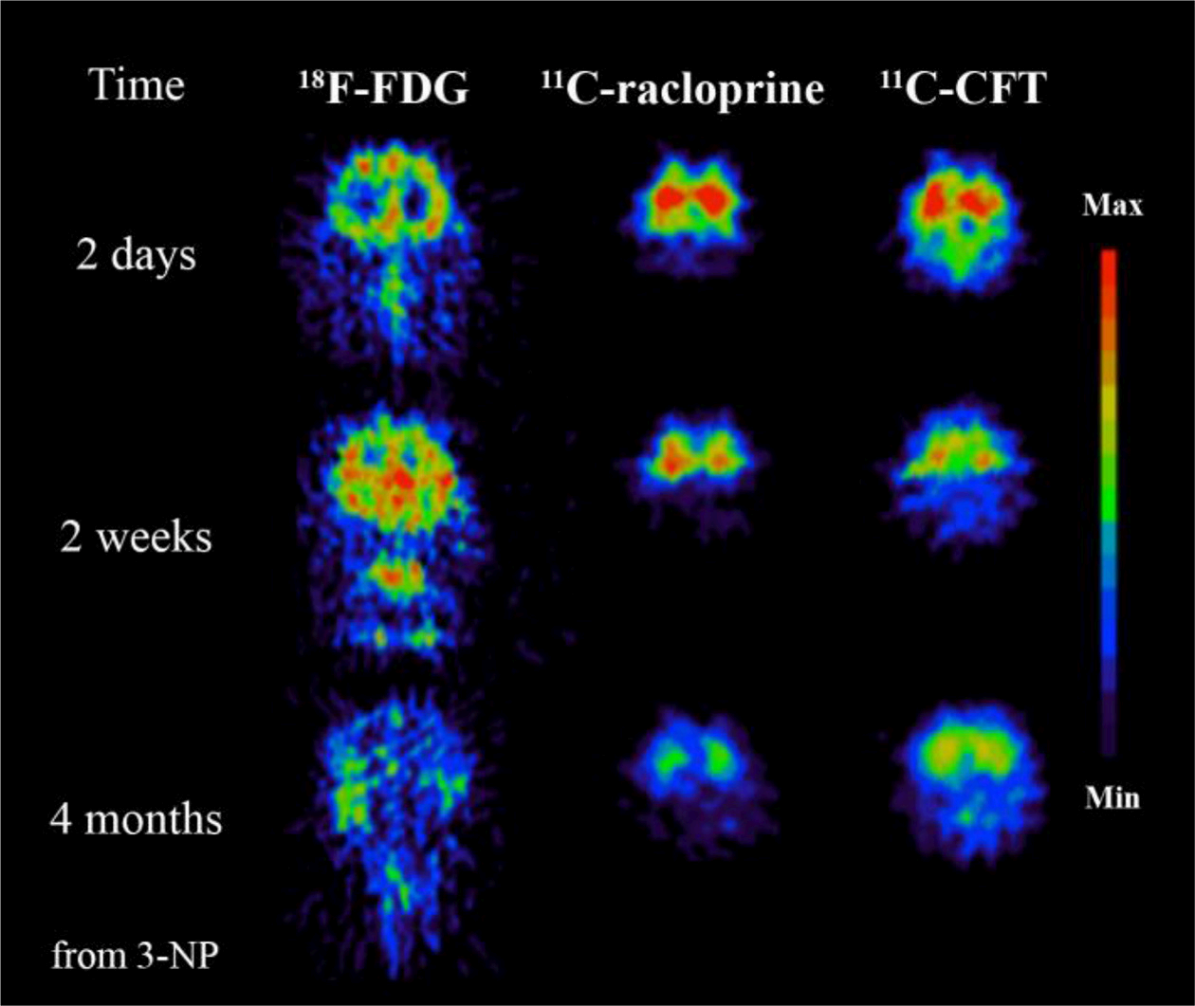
3-Nitropropionic acid (3-NP, a naturally occurring plant toxin and mycotoxin) could be involved to the development of MS. This study demonstrates the advantages of PET imaging where specific tracers can be used to reveal different time dependent neurochemical processes. In this case significant decrease of glucose metabolism imaged by 18F-FDG, decrease of dopamine D2 receptor function imaged by 11C-raclopride and decrease of dopamine transporter function imaged with 11C-CFT follow after 3-NP administration. Modified from Brownell et al. (2004).
